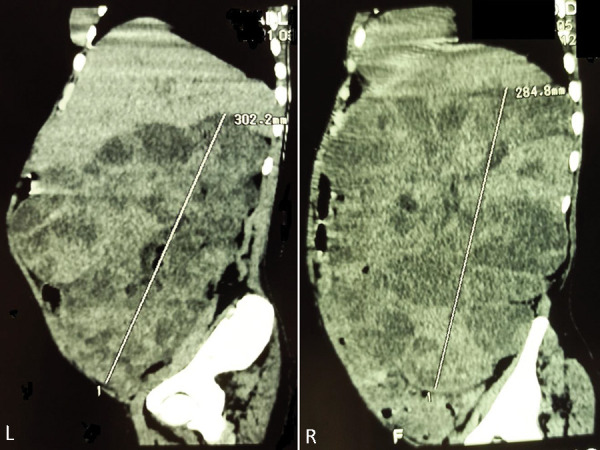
Sagittal CT scan showed two large kidneys measuring 196 × 132 × 284 mm on the right and 214 × 125 × 302 mm on the left with budding and heterogeneous masses with mixed matrix (tissue, fluid, and fat) corresponding to angiomyolipoma.
